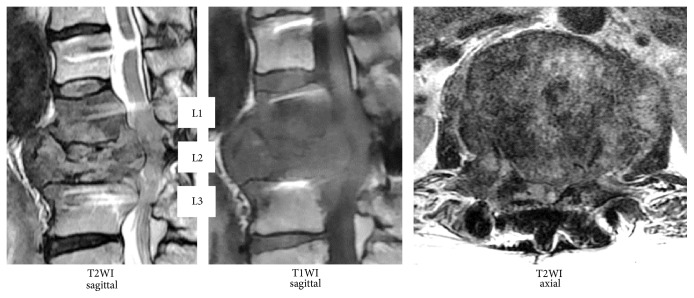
MRI findings. Brightness changes in the L1, L2, and L3 vertebral bodies and compression of the spinal canal by the tumor at the same level.
